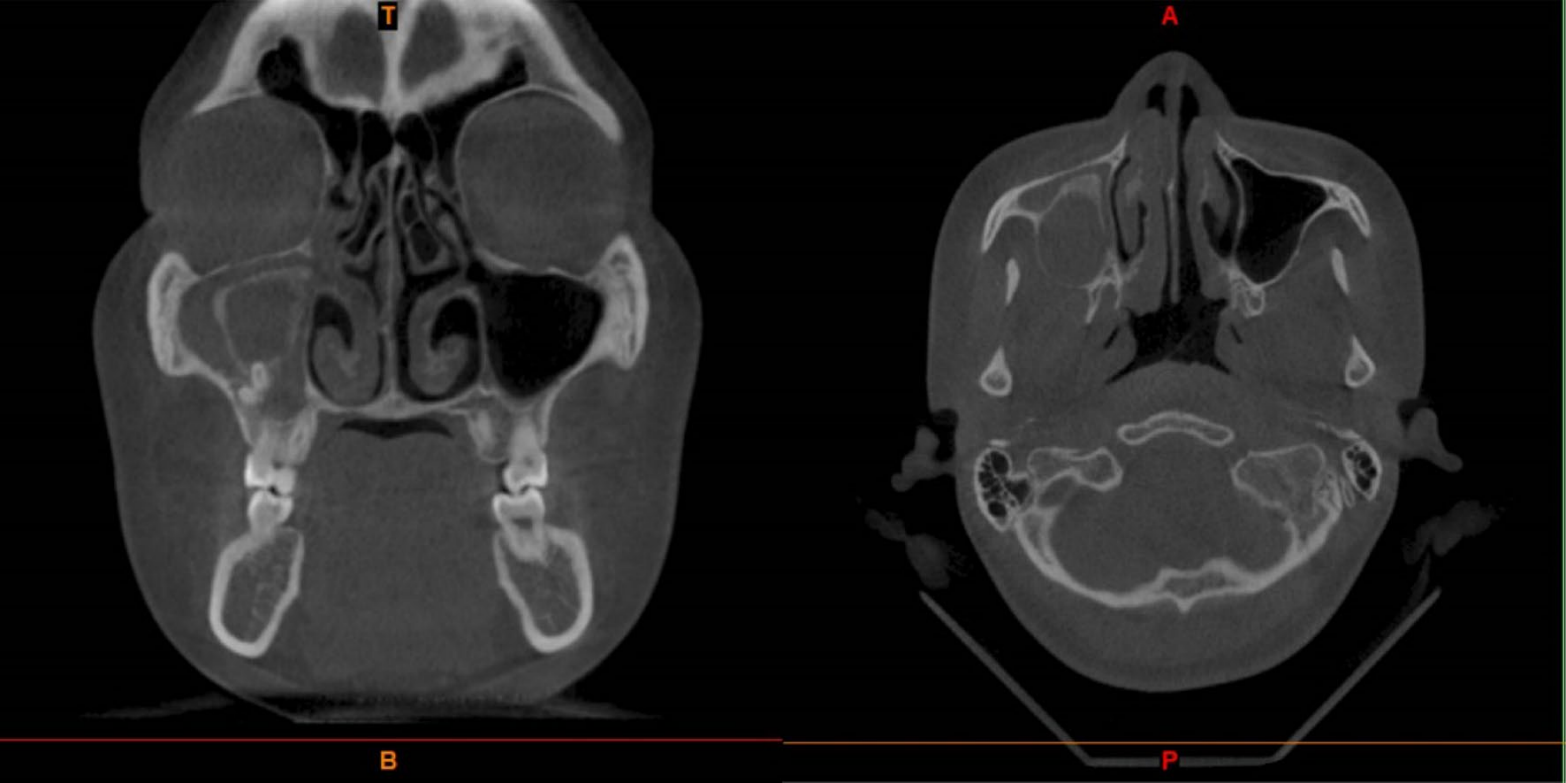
Preoperative Computed tomography showing large cyst in the right maxillary sinus with CMS.
